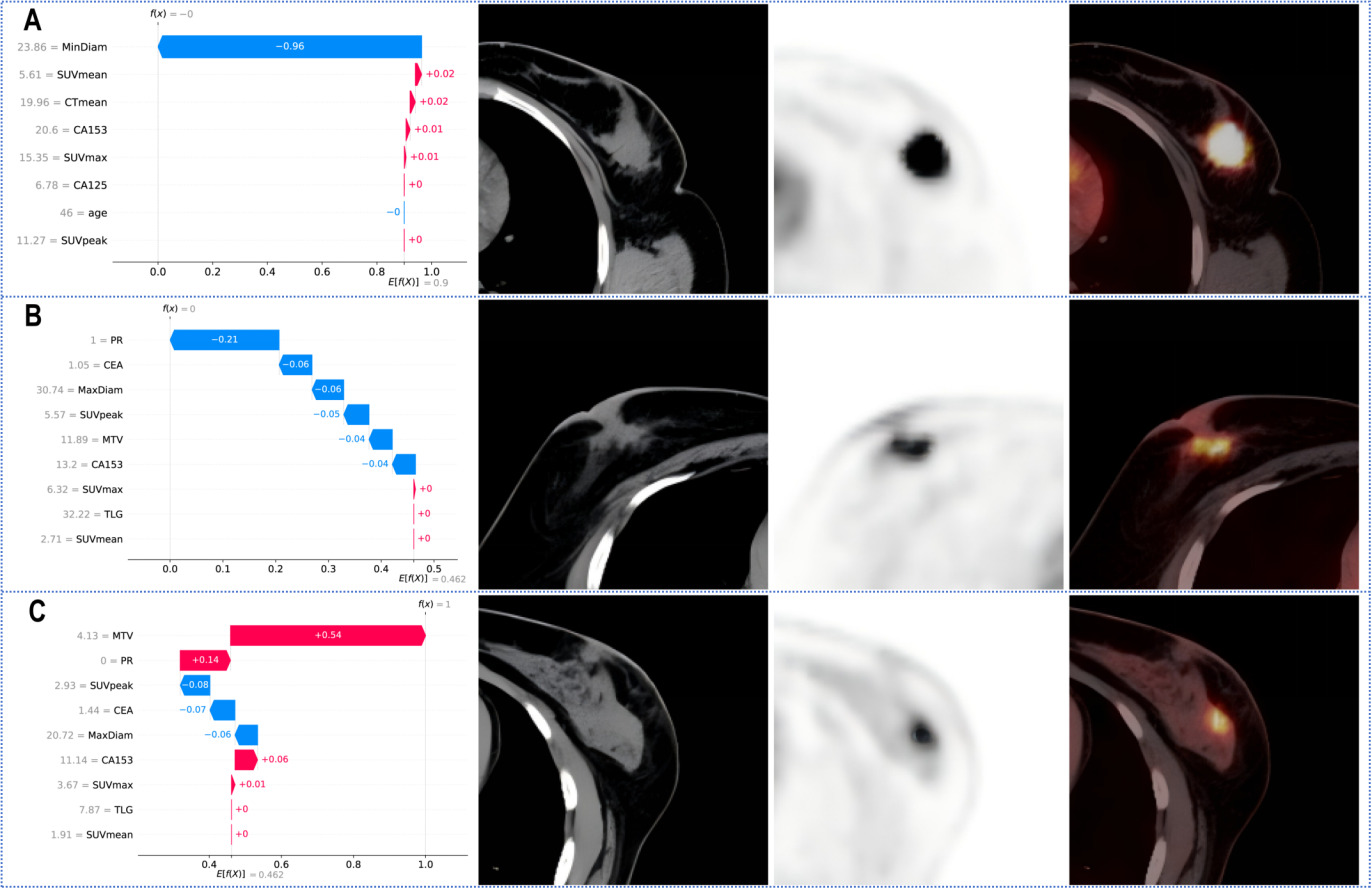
SHAP waterfall plot for predicting (A) HER2-zero, (B) HER2-low, and (C) HER2-positive tumours. PR, progesterone receptor; SUVmax, maximum standardized uptake value; SUVmean, mean standardized uptake value; SUVpeak, peak standardized uptake value; MTV, metabolic tumour volume; TLG, total lesion glycolysis.
